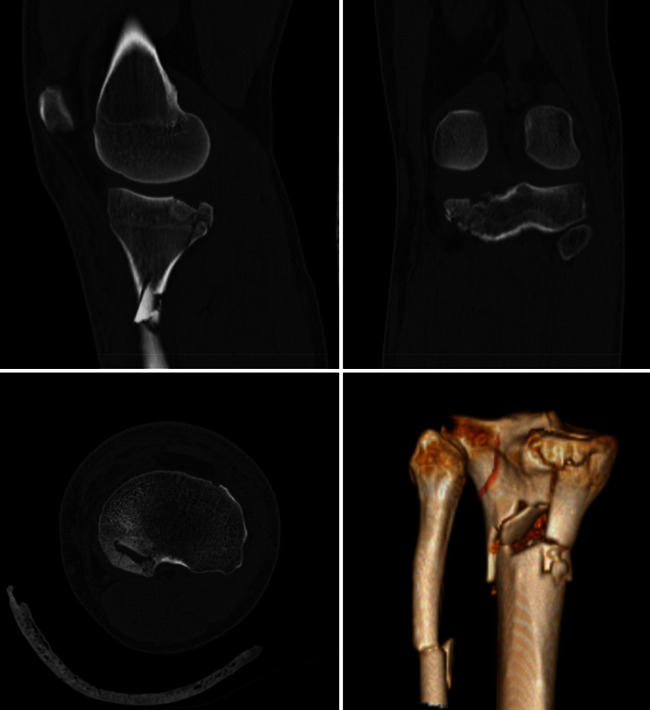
Pre-operative computed tomography and 3D reconstruction.
